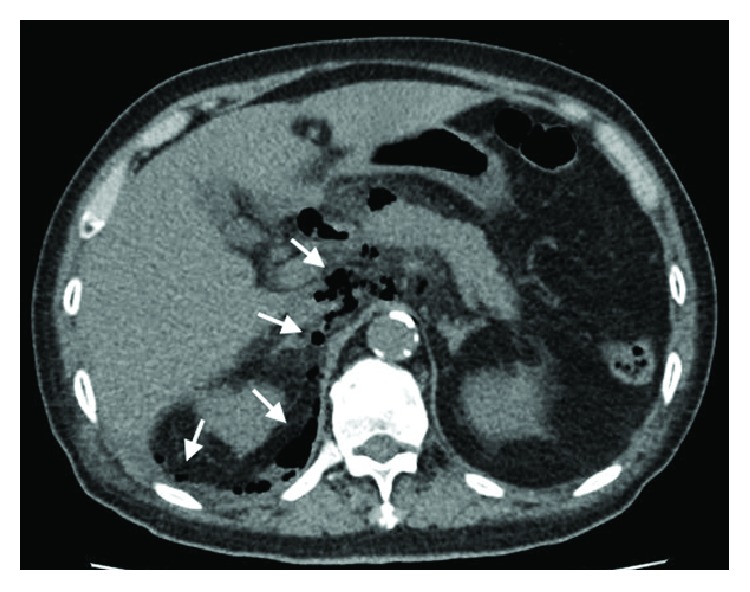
CT scan of abdomen at the level of the pancreas showing pneumoperitoneum with retroperitoneal air involving the right perinephric region.
